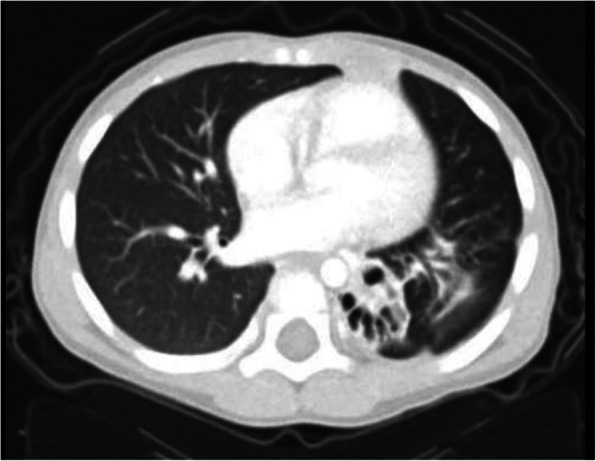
Chest CT showed a significant damage of the affected segment
